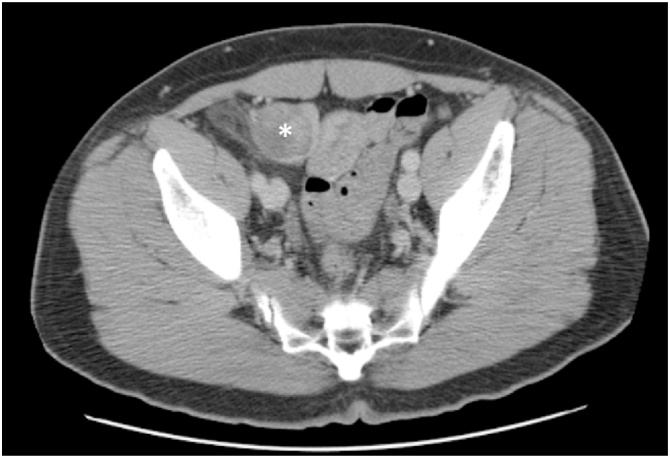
Asterisk represents Computed Tomography (CT) findings of a solid mass in the retroperitoneum, proximal to the inguinal canal.
